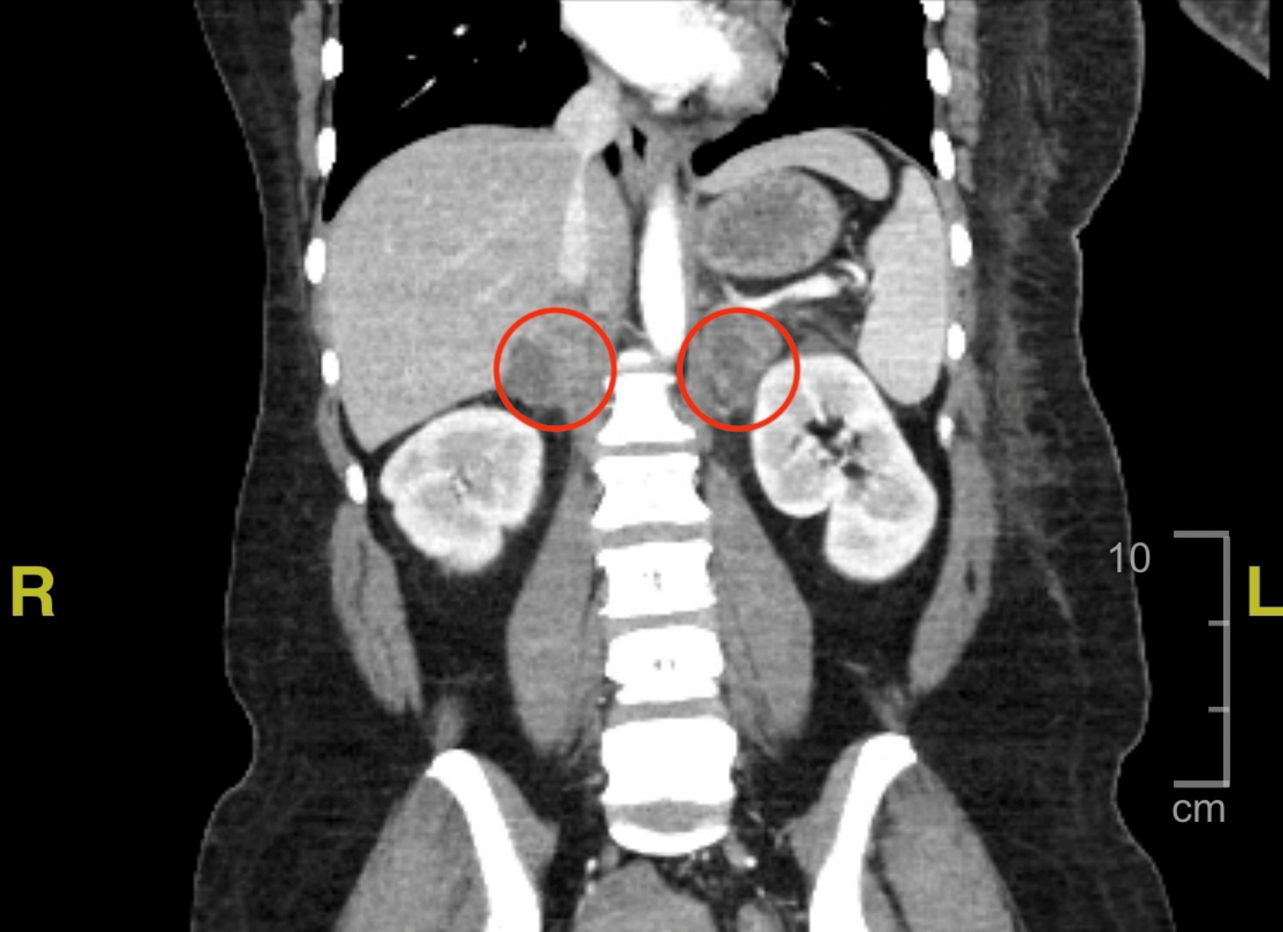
Computed tomography (CT) of the abdomen/pelvis with contrast demonstrating enlarged adrenal glands bilaterally Left red circle: right adrenal gland measuring 5.3 x 2.5 cm. Right red circle: left adrenal gland measuring 5.6 x 2.9 cm
